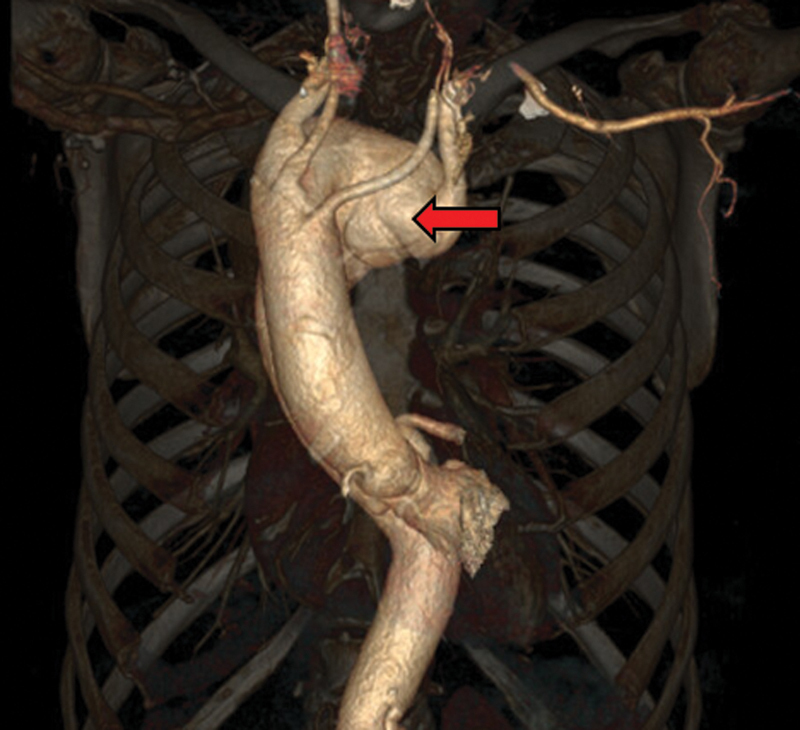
Reformatted preoperative three-dimensional computed tomography scan demonstrating a Kommerell's diverticulum (red arrow) associated with a right-sided aortic arch.
